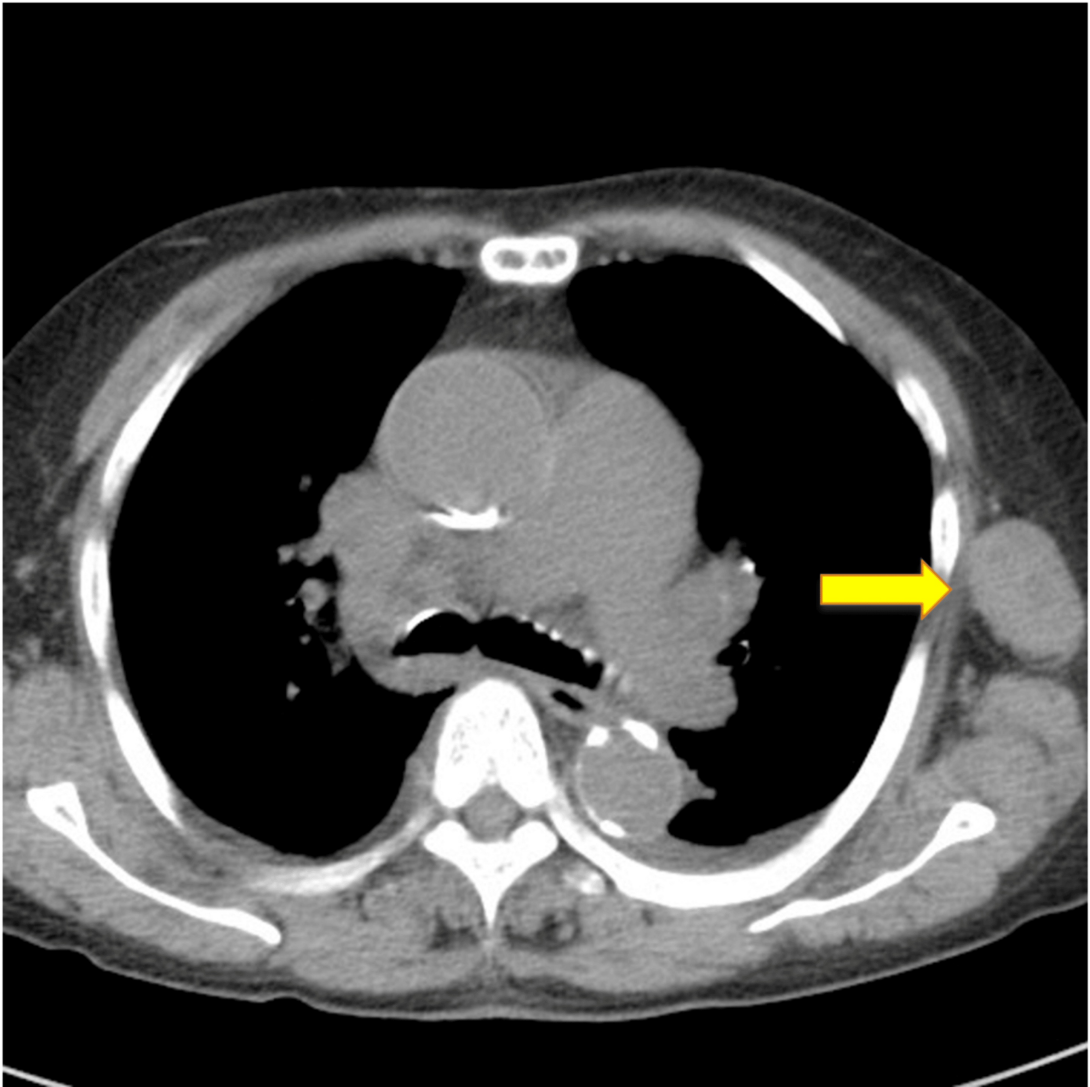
Plain chest computed tomography (CT) Swelling of the left axillary lymph nodes were observed.
